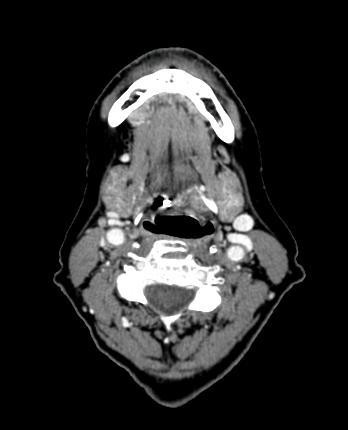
Post contrast axial CT. Post contrast axial CT scan demonstrating a heterogeneous, well circumscribed lesion in the left pre-epiglottic space abutting the posterior surface of the hyoid bone at the junction of the body and the cornua.
